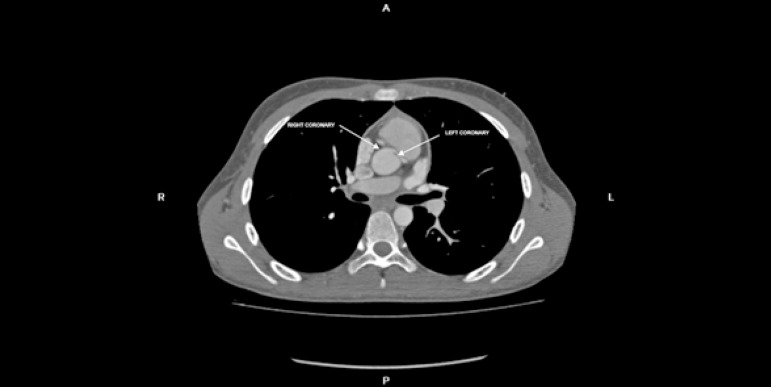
CT angiography shows anomalous origin of the left coronary artery arising
